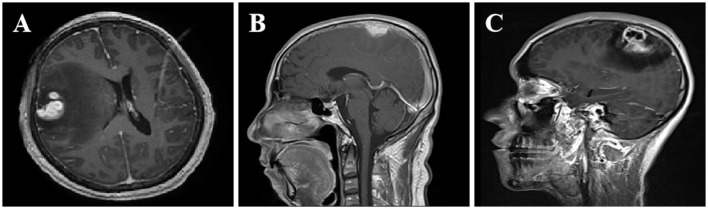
Enhanced MRI imaging features of CSG. MRI imaging features of SCG. (A) After contrast enhancement, the lesions in the right frontotemporal lobe showed nodular enhancement. (B) The right frontal lobe lesions demonstrated homogeneous and prominent enhancement upon contrast-enhanced scan, accompanied by thickening and enhancement of the adjacent meninges. (C) The lesions in the left parietal lobe showed uneven ring-like enhancement after contrast enhancement, accompanied by thickening and enhancement of the adjacent meninges.
